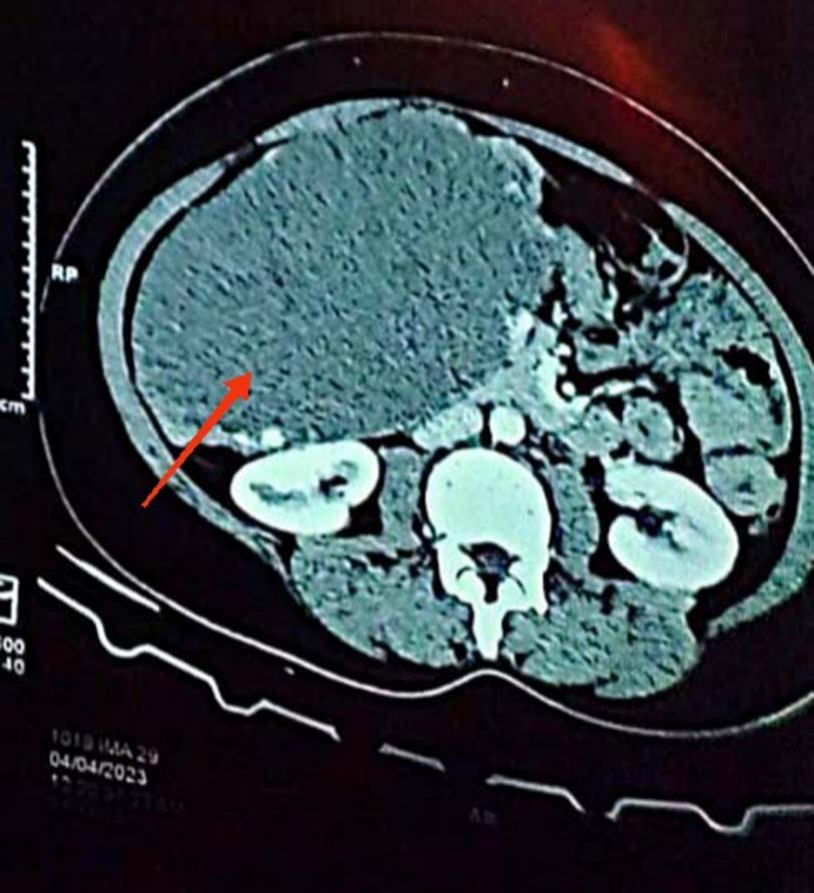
Computed tomography of abdomen showing hypodense lesion (hemangioma) in liver by red arrow.
